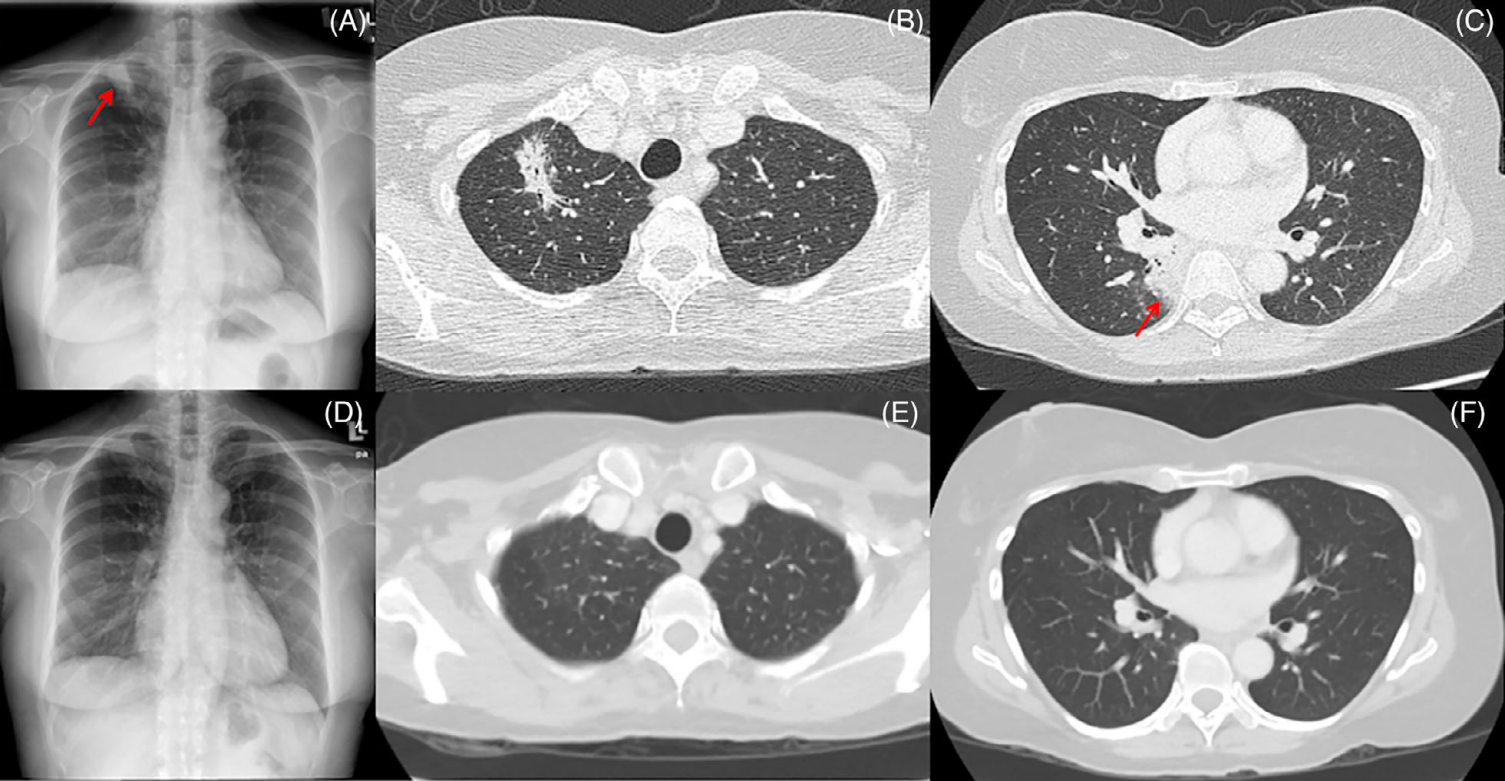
Chest radiograph (A) on initial presentation showed air space opacity seen in the right lung apex (red arrow). Axial view of the computed tomography (CT) thorax showed consolidation with air bronchogram at the right upper lobe (B) and superior segment of the right lower lobe (C) (red arrow). Chest radiograph (C) after 3 months of corticosteroids therapy revealed improvement in the right apical lung consolidation. Axial view of the CT thorax (E and F) showed resolution of previously reported multifocal lung consolidations
